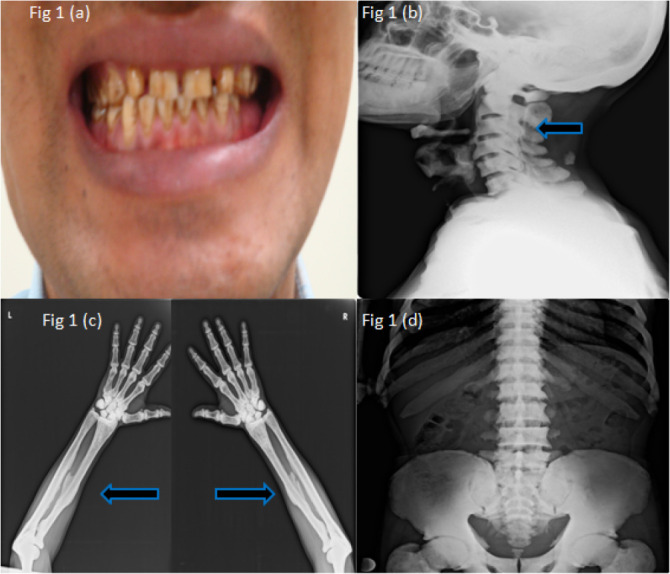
(a) Teeth showing dark brown streak and discoloration with pitted and rough enamel. (b) X-ray of skull and cervical spine showing osteosclerosis and the posterior longitudinal ligament ossification with calcification of thyroid and cricoid cartilage. (c) X-ray of both forearms showing interosseous membrane calcification. (d) X-ray of the pelvis showing osteosclerosis of the vertebral column, exuberant calcification of pelvis and greater trochanter along with prominent hypertrophic spurring at the acetabular margins.
